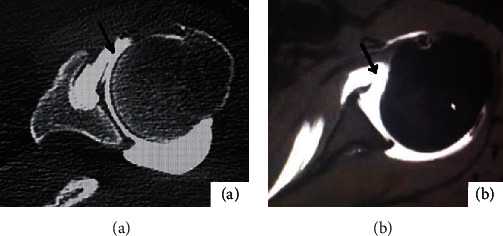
Female, 24 years old, anterior dislocation of left shoulder for 5 months. (a) shows the bone window image on the horizontal axis of CTA of the left shoulder; as shown by the black arrow, anterior inferior glenohumeral ligament was torn, and part of contrast medium was leaked. (b) shows the T1WI-FS image in the transverse axis of the MRA of the left shoulder. The black arrow shows that the anterior ligament of the inferior glenohumeral ligament is torn, and the broken end is retracted.
